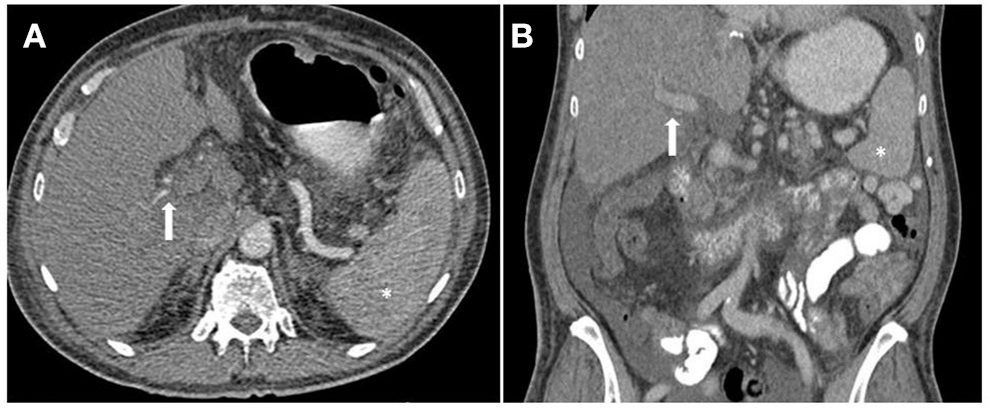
Computed tomography (CT) image of patient with Seventh-Day Syndrome. Axial (A) and coronal (B). (A) The arterial phase axial image on the left demonstrates a patent right and left hepatic artery (arrow) and a relatively hypoenhancing liver in comparison to the spleen (*). (B) The coronal image in the portal venous phase demonstrates a patent portal vein.
